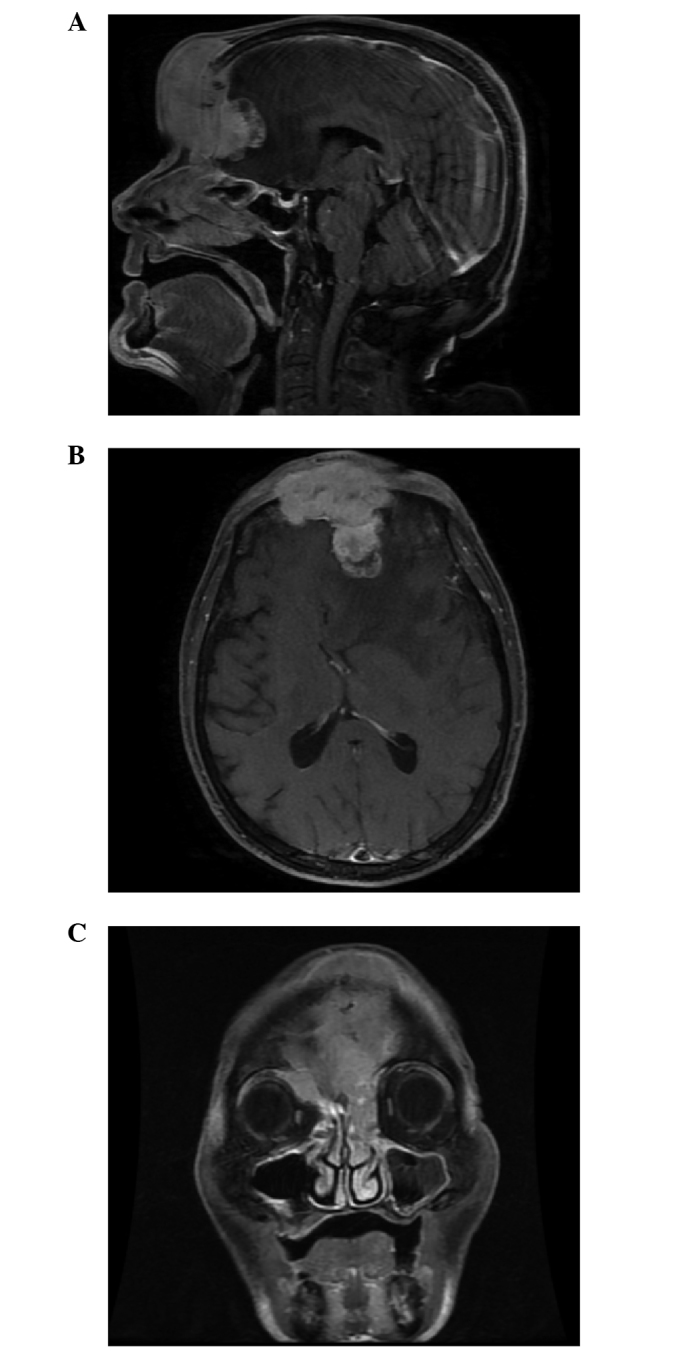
Enhancement of the magnetic resonance imaging (MRI) scan revealing an extensive area of abnormal tissue intensity involving the (A) left frontal sinus, (B) frontal lobe and (C) ethmoid sinus.
